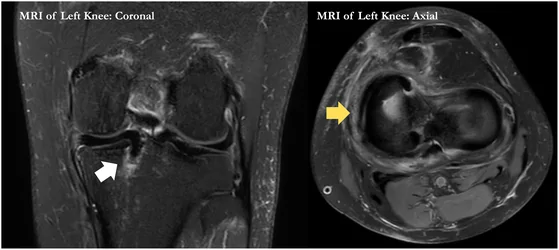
Two‐month postoperative magnetic resonance imaging of the left knee following medial meniscal allograft transplantation, showing appropriate graft alignment. The white arrow indicates secure posterior root fixation, and the yellow arrow denotes a well‐positioned meniscal allograft without evidence of extrusion. (MRI, magnetic resonance imaging.)
